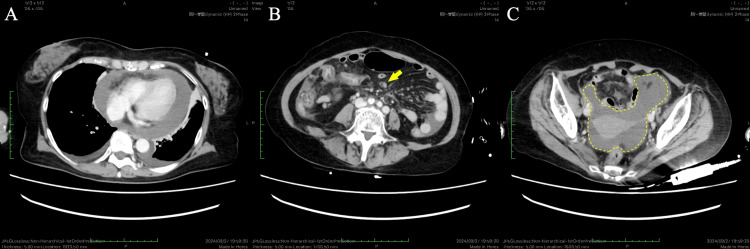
CT images at diagnosis Chest CT (A) reveals massive pericardial effusion and mild bilateral pleural effusion. Abdominal CT (B, C) reveals peritoneal dissemination (arrow) and accumulation of ascites in the pelvic cavity (dotted circle).
